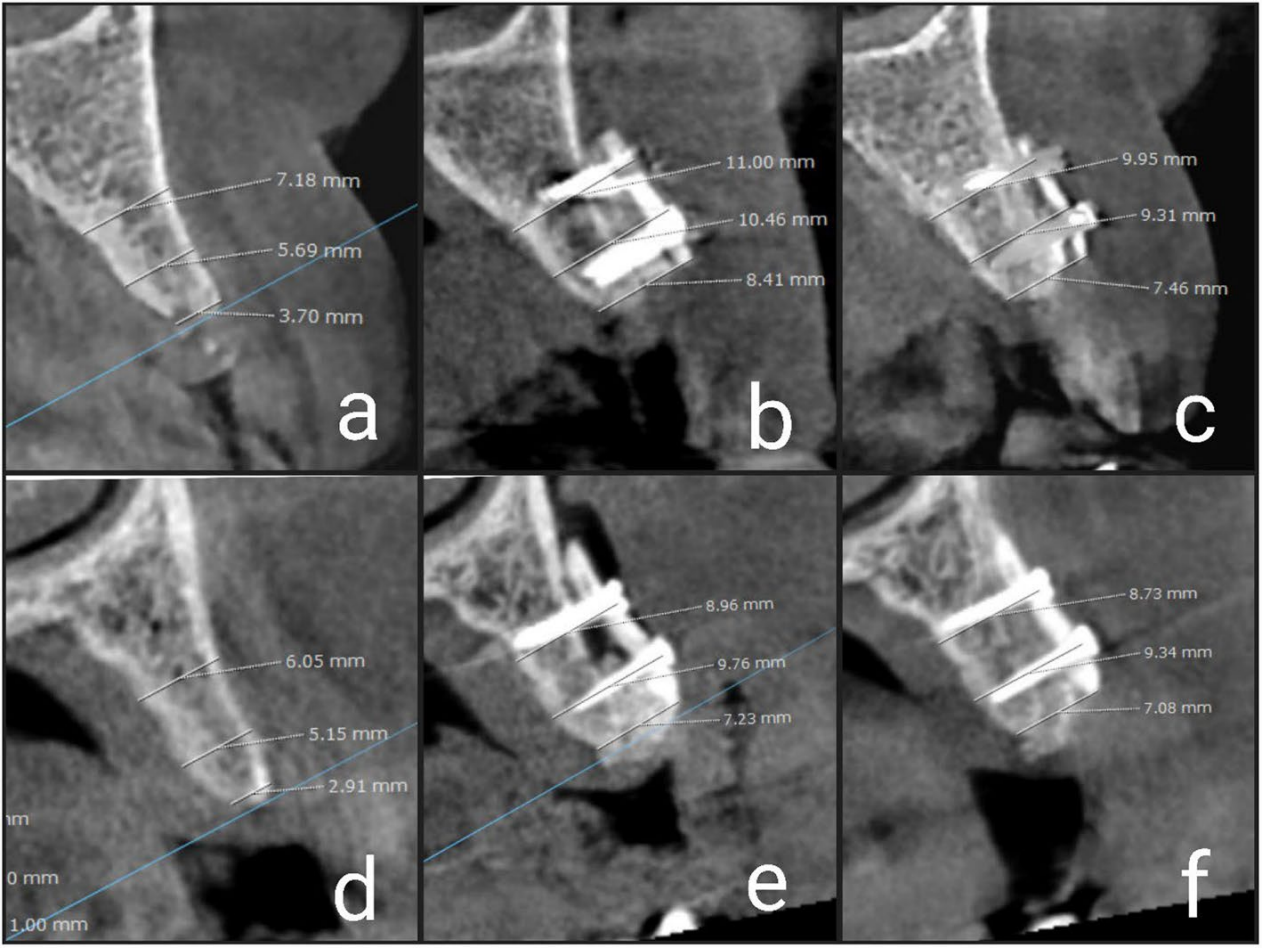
Showing radiographic evaluation: BST (a-c); (a) Preoperative; (b) Immediate postoperative; (c) After 6 months. TST (d-f); (d) Preoperative; (e) Immediate postoperative; (f) After 6 months
